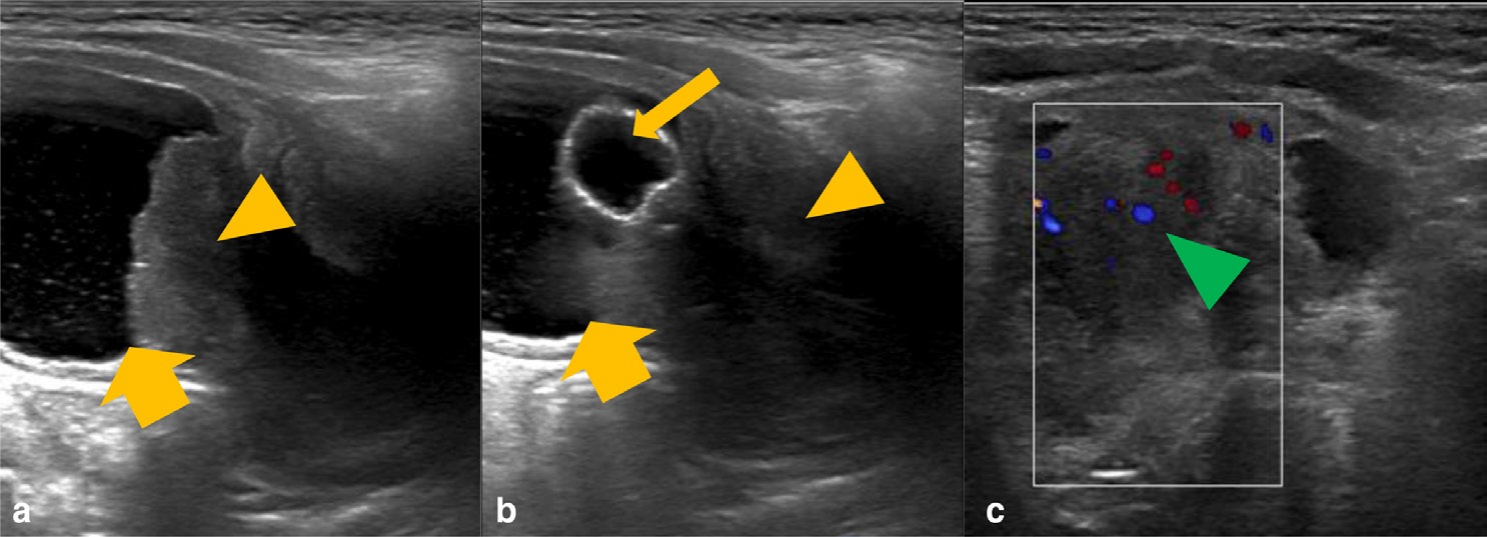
Grayscale ultrasound images of the bladder and prostate gland (A and B) with further colour doppler ultrasound (C). There is a heterogeneous mass in the expected location of the prostate gland (orange arrowhead), which is invaginating into the urinary bladder base (thick orange arrow). There is evidence of increased internal vascularity (green arrowhead). The urinary catheter courses through the mass and the balloon is within the urinary bladder (thin orange arrow).
